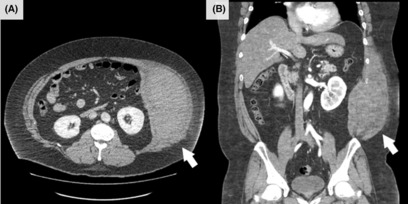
Contrast‐enhanced computed tomography of the abdomen showing the abdominal internal oblique muscle hematoma (arrow) (A: axial view, B: coronal view).
